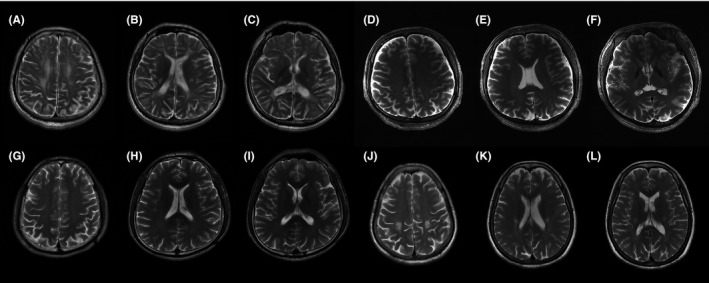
T2‐weighted MRI on the same three levels (centrum semiovale, corona radiate, and internal capsule) of patients with GLD. A, B and C, MRI of proband 5 at his 21 showed partly confluent hyperintense areas in white matter near the lateral ventricle and posterior limbs of internal capsule. D, E and F, MRI of proband 6 at his 28 showed no visible abnormality. G, H and I, MRI of proband 7 at his 28 showed no visible abnormality. J, K and L, MRI of proband 8 at her 46 showed symmetric hyperintense in bilateral corticospinal tracts
